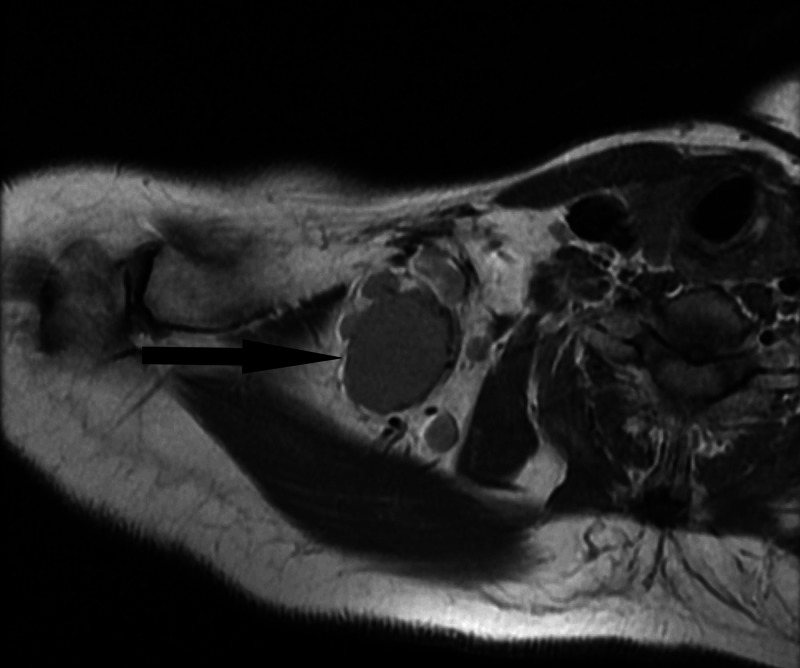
Right brachial plexus proton density-weighted MRI showing a 3.3cm by 2.6cm mass.
